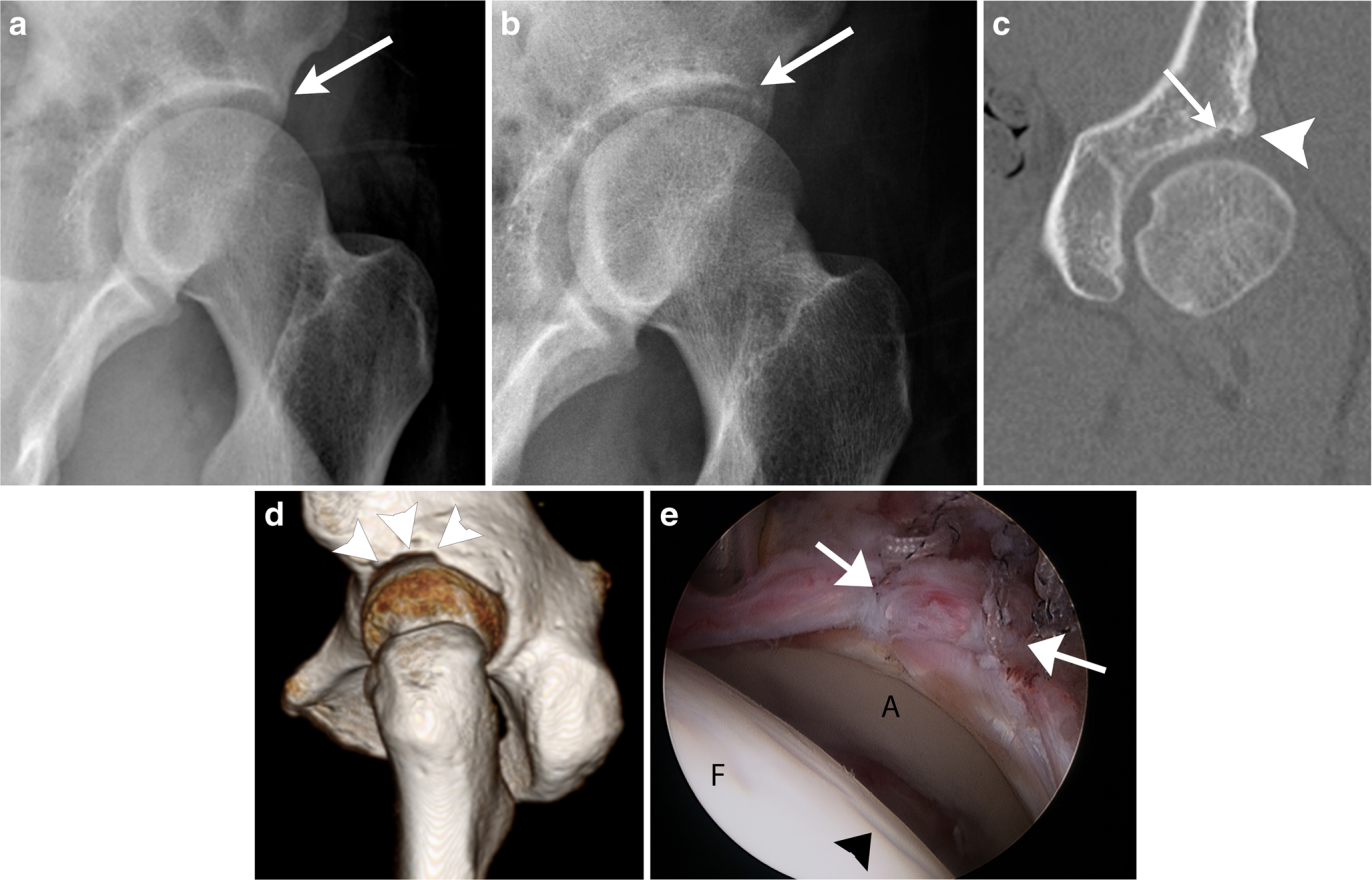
Pincer lesion before and after surgery in a 32-year-old woman. a Preoperative false profile view shows prominent contour of the anterior acetabulum (*arrow*). b Postoperative false profile view shows subtle bone resection (*arrow*). c Coronal postoperative CT shows the bone resection (*arrowhead*) and a lucent track (*arrow*) where a bioabsorbable suture anchor was placed as part of labral repair. d 3D postoperative CT best shows the extent of bone resection (*arrowheads*). e Arthroscopy picture shows labral repair (*arrows*) after rim trim.* A* articular surface of the acetabulum,* F* femoral head.* Arrowhead* points to articular cartilage damage, probably related to the arthroscopic instruments
